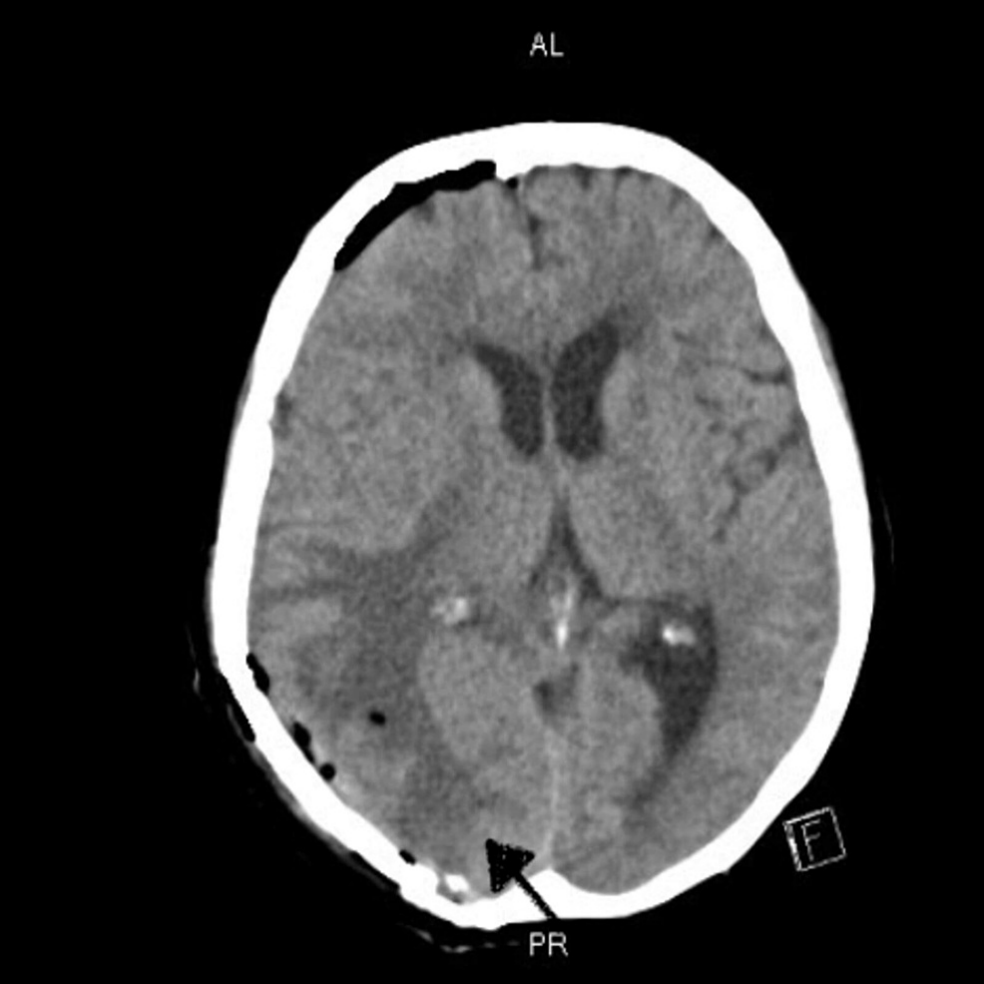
Post-Surgery CT Scan of the Head (Axial View) Without Any Evidence of Mass, Mass Effect, Midline Shift or Hemorrhage
